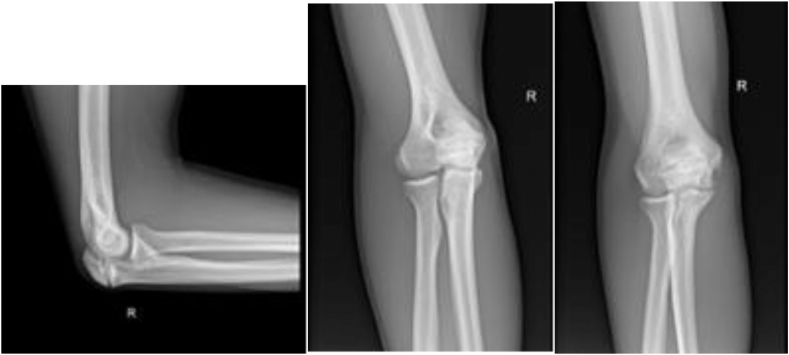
Initial injury plain films: transverse olecranon stress fracture with sclerotic fracture edges. Well-maintained ulnohumeral and radiocapitellar joint spaces.
